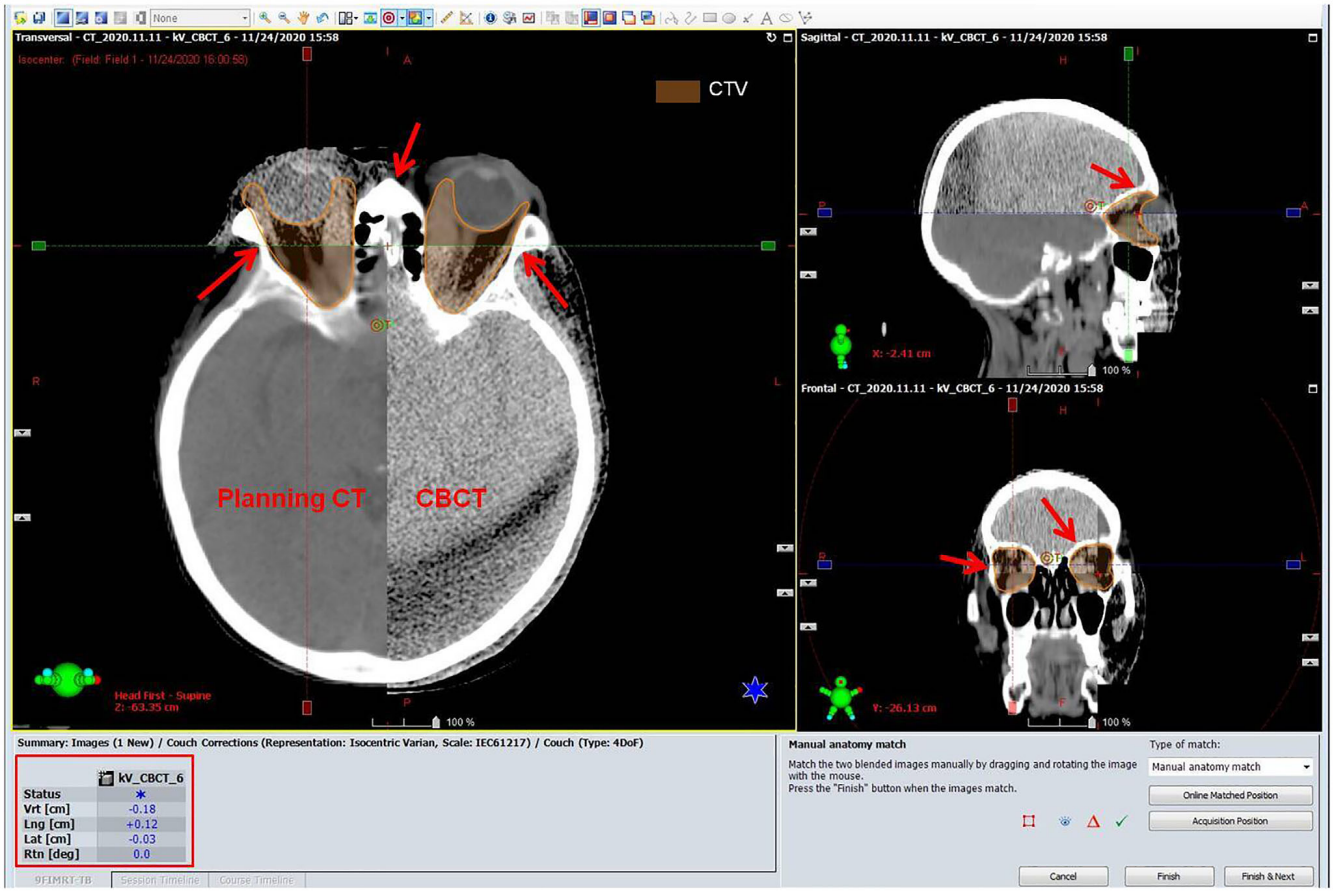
Setup errors in cone beam CT (CBCT) bony alignment correction in a patient with moderate to severe thyroid‐associated ophthalmopathy (TAO).
